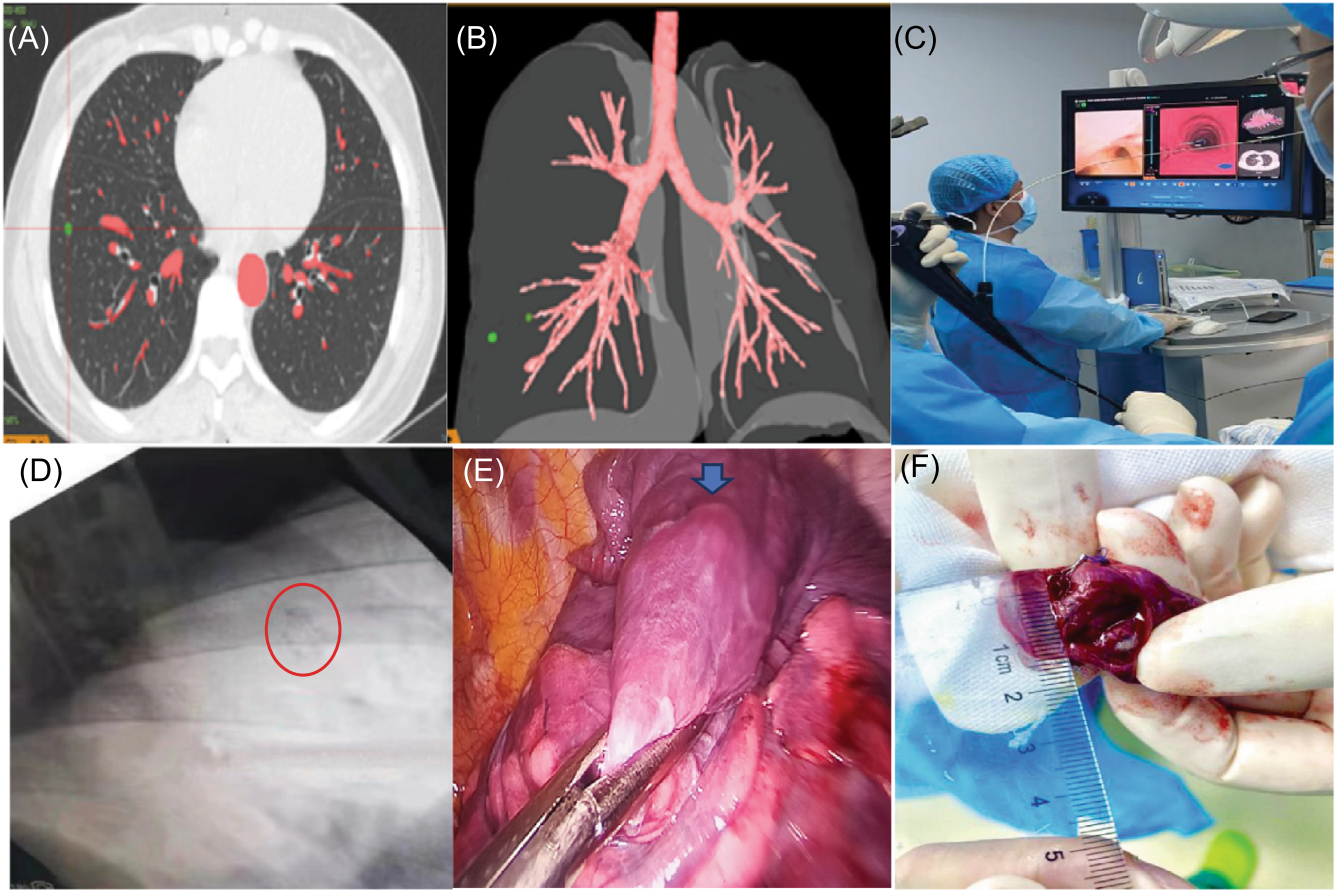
The following photos are from the Third Affiliated Hospital of Kunming Medical University: (A) preoperative CT images, (B) 3D model of Lung‐pro reconstruction; (C) marker placement procedure, (D) DR confirms surgical marker location; (E) markers seen intraoperatively (at arrows); (F) resected markers and nodules.
